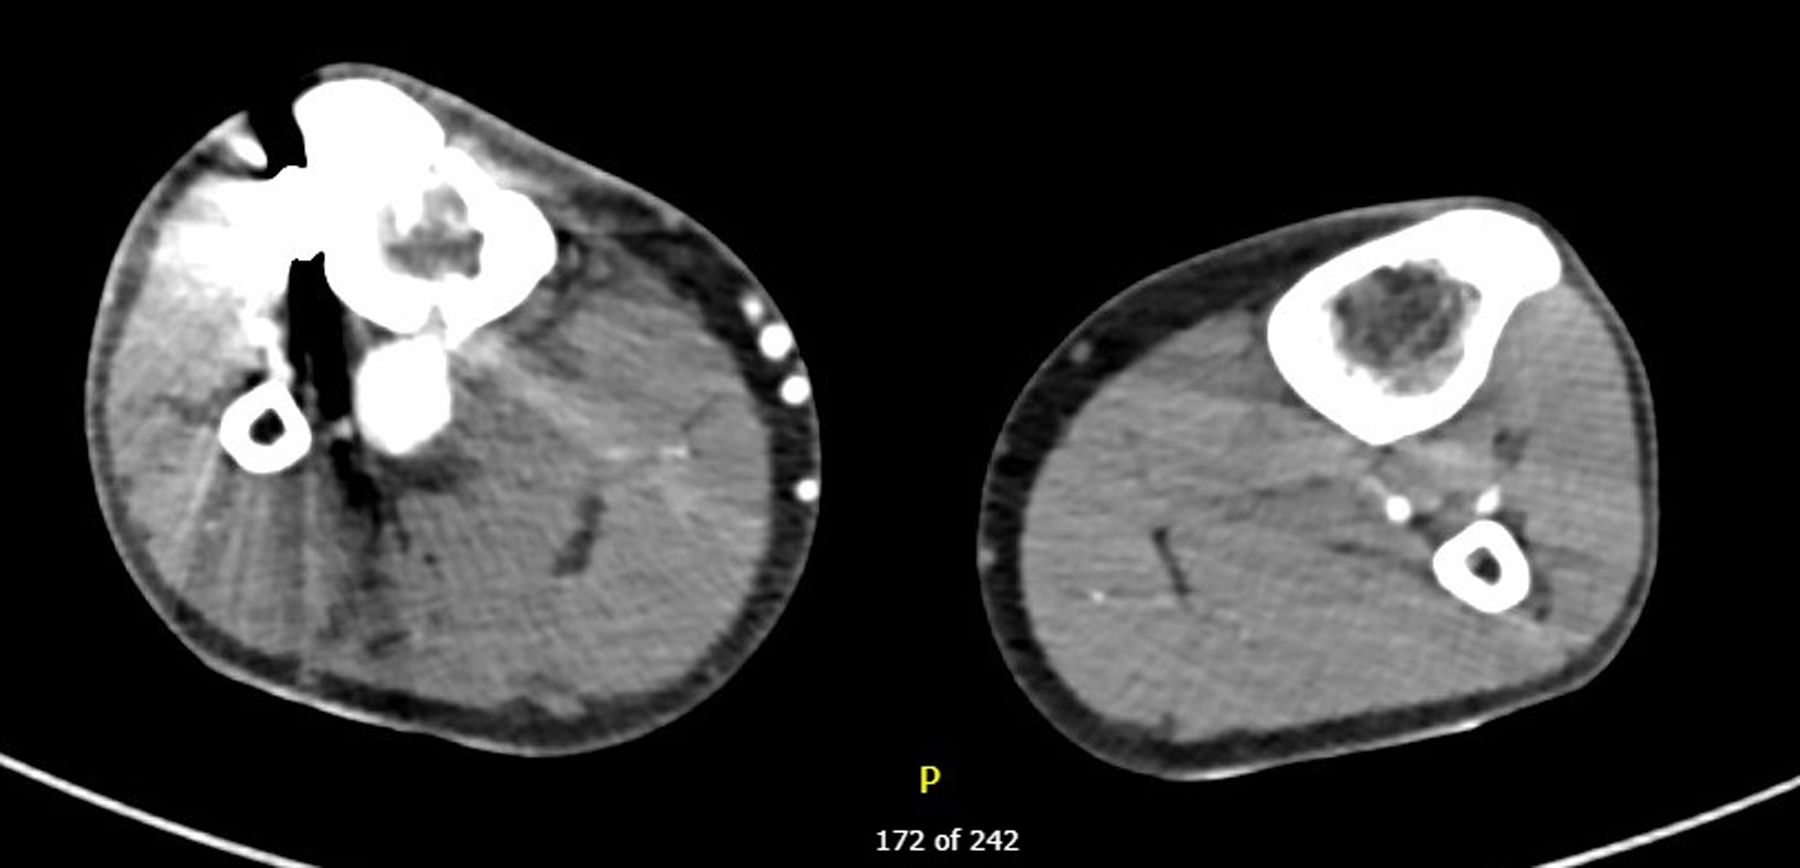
CT scanner in arterial phase where the contrast product passes into arteriovenous fistula.
